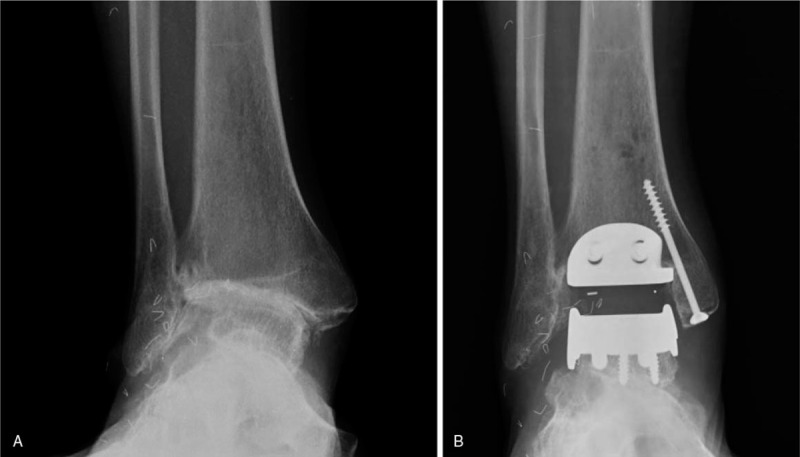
Weight-bearing anteroposterior radiographs of a 50-year-old woman. Preoperative radiograph indicating 14° of ankle-incongruent valgus deformity due to growth arrest in the lateral aspect of the distal tibia (A). Postoperative radiograph (B).
